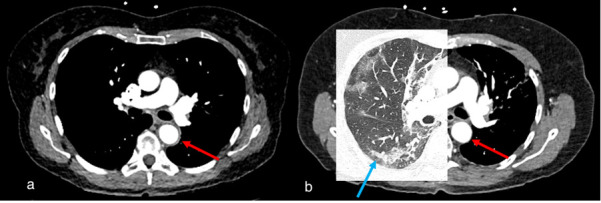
Case 1, a 67-year-old female. (a) Initial chest CT to clarify pulmonary embolism or post-COVID-19 findings showed concentric wall thickening of the thoracic aorta (red arrow), indicating large vessel vasculitis. No pulmonary infiltrates or post-COVID-19 findings were observed. (b) Follow-up computed tomography 2 months later showed indeterminate appearance of recurrent COVID-19 pneumonia (blue arrow) and regressed arterial wall thickening (red arrow).
